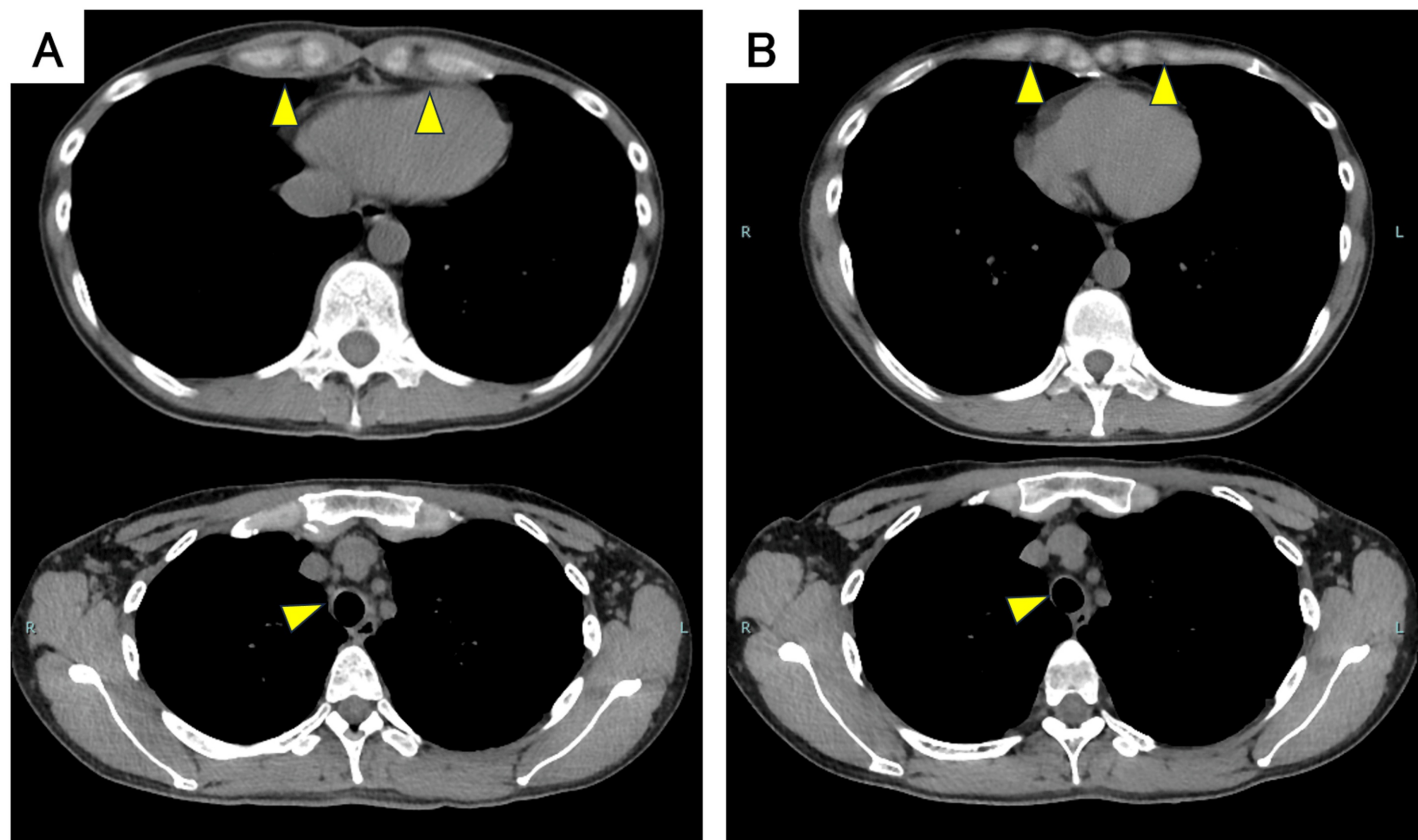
Comparison of chest CT findings (A) before and (B) two months after treatment. These showed a reduction in the soft tissue shadow thickening around the costal cartilage and in the thickening of the tracheal and bronchial walls.
